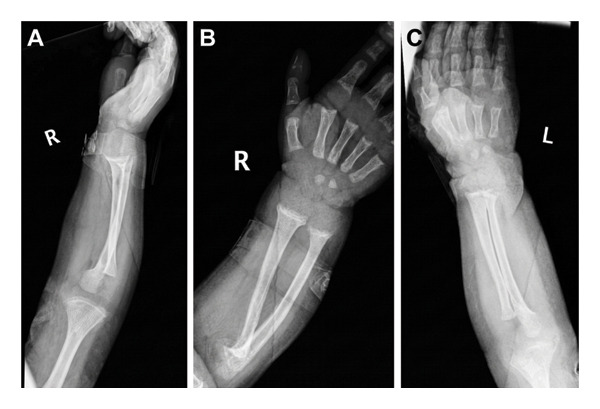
Baseline wrist and forearm radiographs of Case 1 demonstrating rachitic changes at presentation. Note: (A–C) Radiographs obtained at 7 months of age demonstrating widening of the distal radial and ulnar growth plates with metaphyseal cupping and fraying, consistent with active rickets. Panel (A) shows a lateral view of the right forearm and wrist, panel (B) shows an anteroposterior view of the right wrist/hand, and panel (C) shows a left wrist/forearm view.
